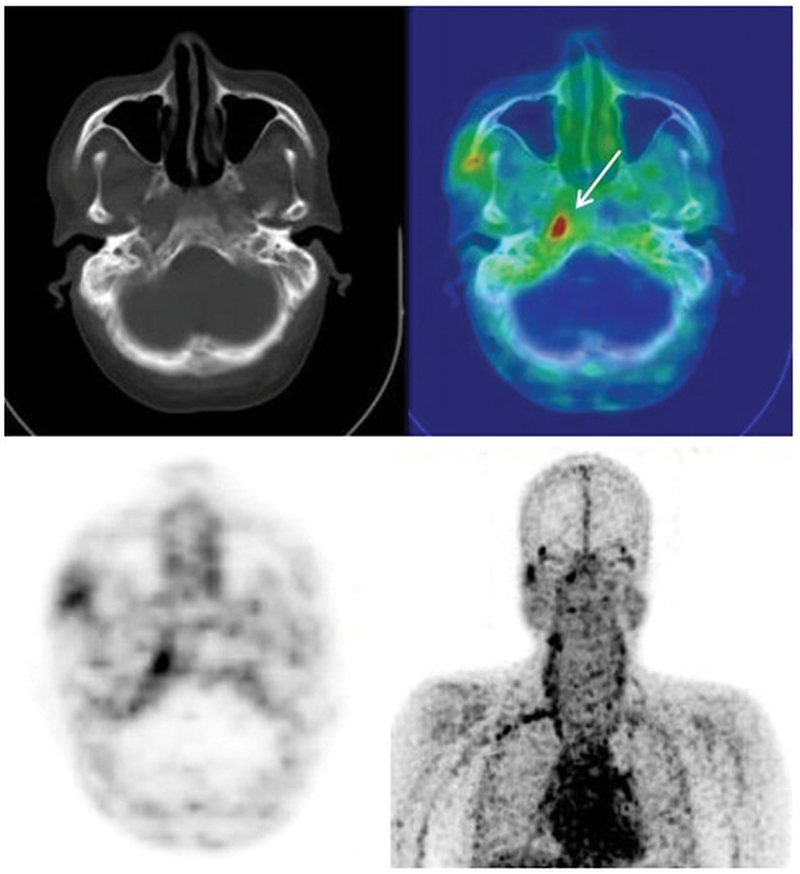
A 46-year-old lady with history of left lateral and central skull base osteomyelitis (SBO), for assessment, Ga-68 citrate positron emission tomography/computed tomography (PET/CT) done showed citrate avid significant enhancing soft tissue thickening in the left carotid space completely encasing the left internal carotid artery, involving the left petrous apex and the left half of clivus.
